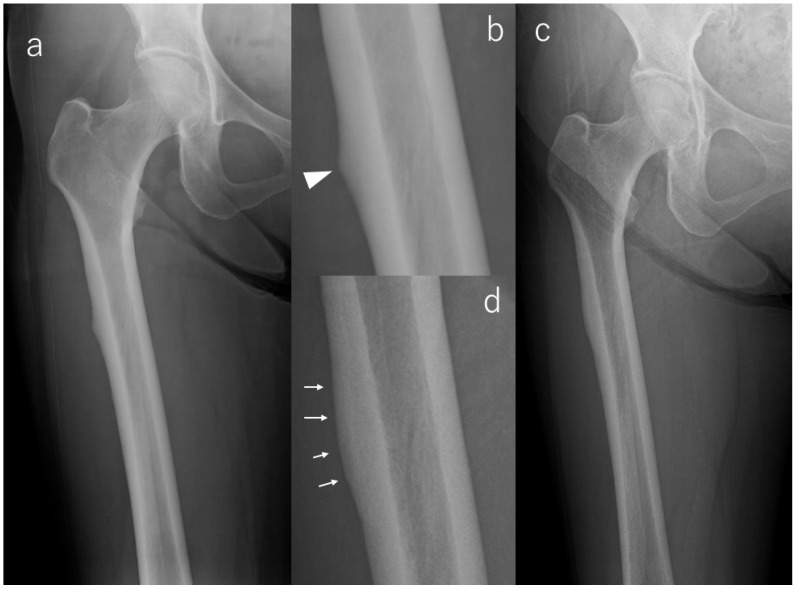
X-rays of Case #3. (a): Radiograph at the time of injury, (b): Enlarged image of a; bump (arrowhead) in the lateral cortex, (c): Radiograph at the last observation, (d): Enlarged image of (c); flattening of beak tip (arrows).
